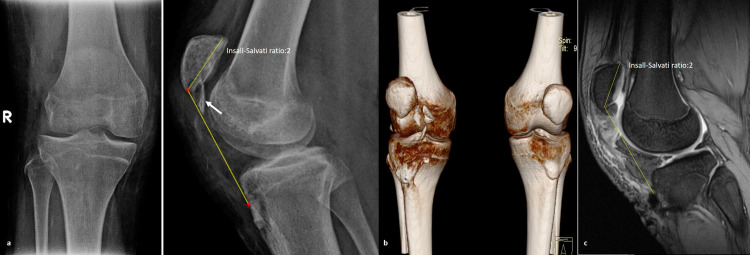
Radiological workup. (a) Anteroposterior and lateral radiographs showing a healed tuberosity fracture with evidence of patella alta (with the knee in 30° of flexion) and ossification of the patella tendon probably due to the previous operation (white arrow); (b) 3D-CT scan of both knees showing a high-riding patella on the right and multiple bone fragments or ossified parts of the tendon; (c) MRI scan of the affected knee demonstrating avulsion of the patella tendon with severe scarring and retraction and obvious patella alta. 3D-CT: three-dimensional-computed tomography
